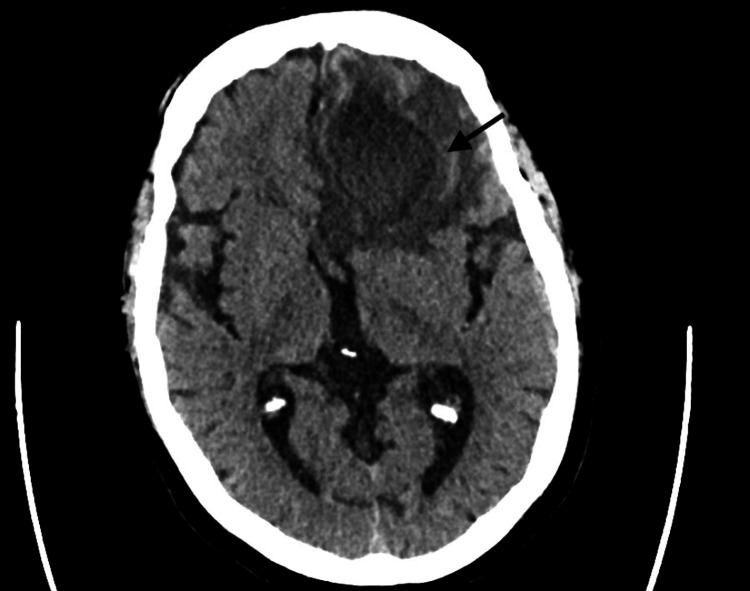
CT of the head without contrast CT of the head without contrast revealed a solitary 3.5 cm mass in the left frontal lobe with vasogenic edema and mass effect upon the anterior horn of the left lateral ventricle.
